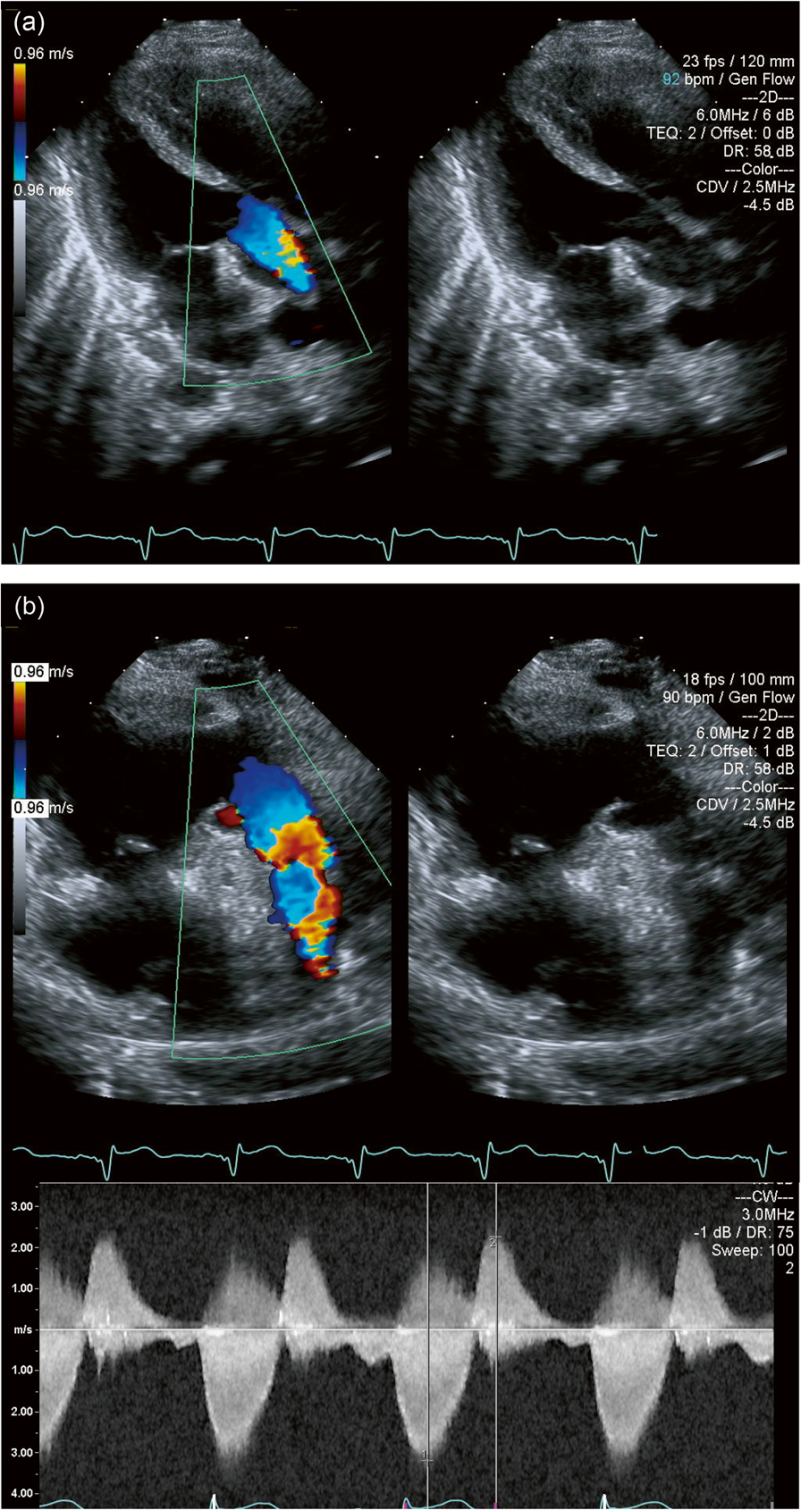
Postoperative echocardiography showing (a) a straight flow of the left ventricular outflow tract without stenosis and (b) mild pulmonary stenosis in the right ventricular outflow tract; peak velocity is 3 m/s
